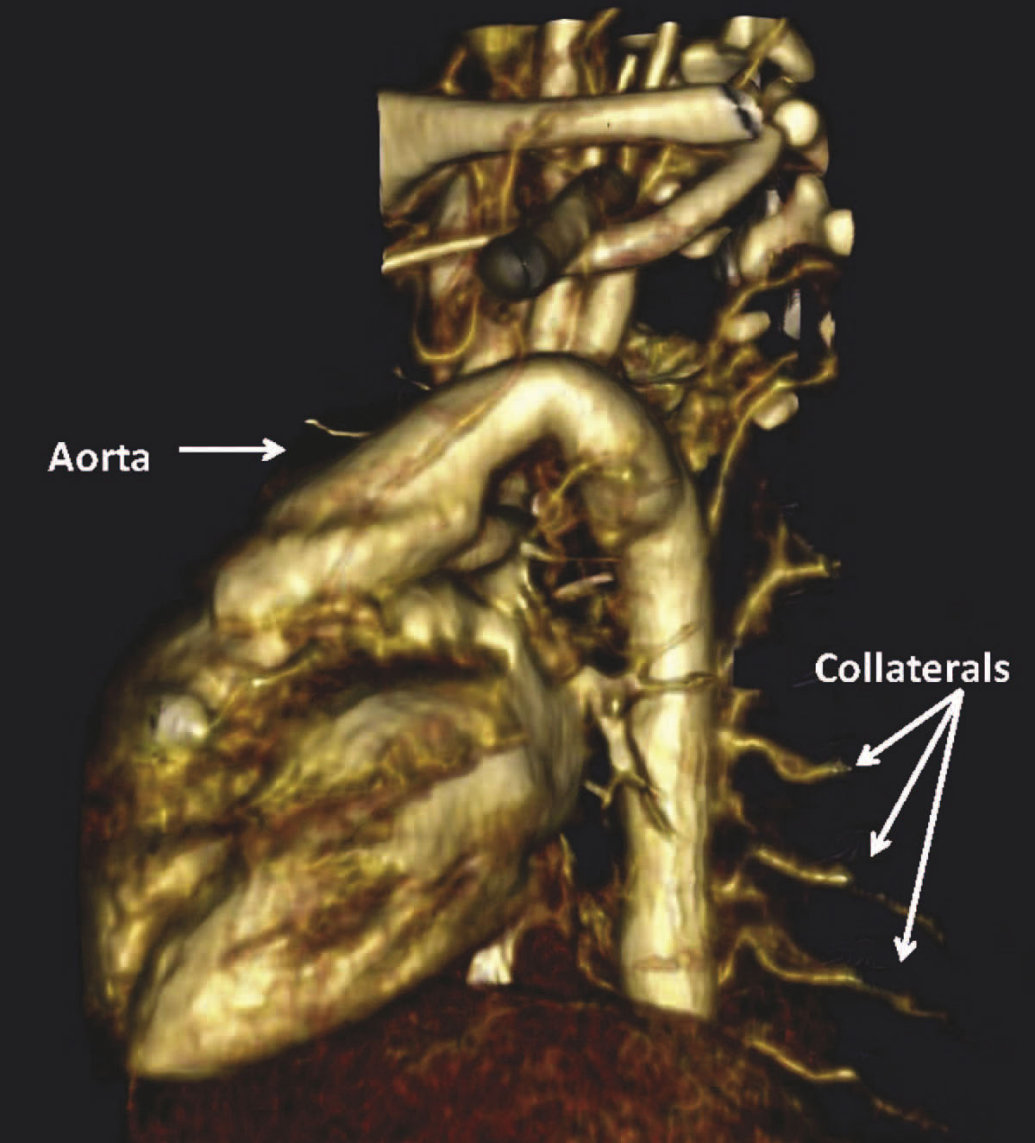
Three-dimensional view on computed tomography angiography showing collateral vessels originating from the aorta and supplying the left lung.
